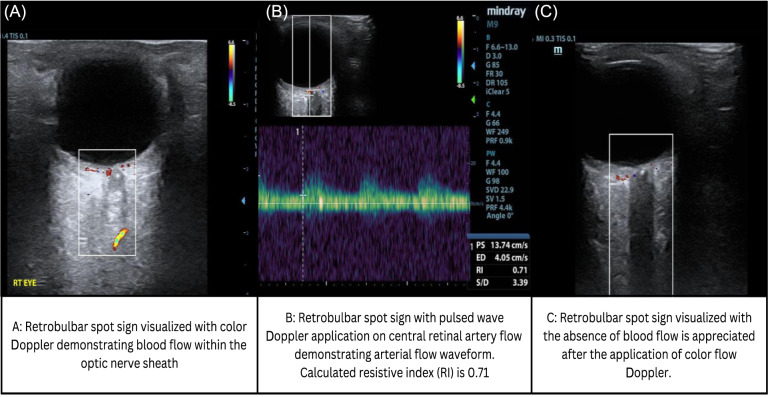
Central retinal artery with acute thrombus and color flow on color Doppler evaluation (A), central retinal artery with increased resistance to flow and an elevated resistive index (B), and absence of central retinal artery color Doppler flow upon repeat evaluation (C).
